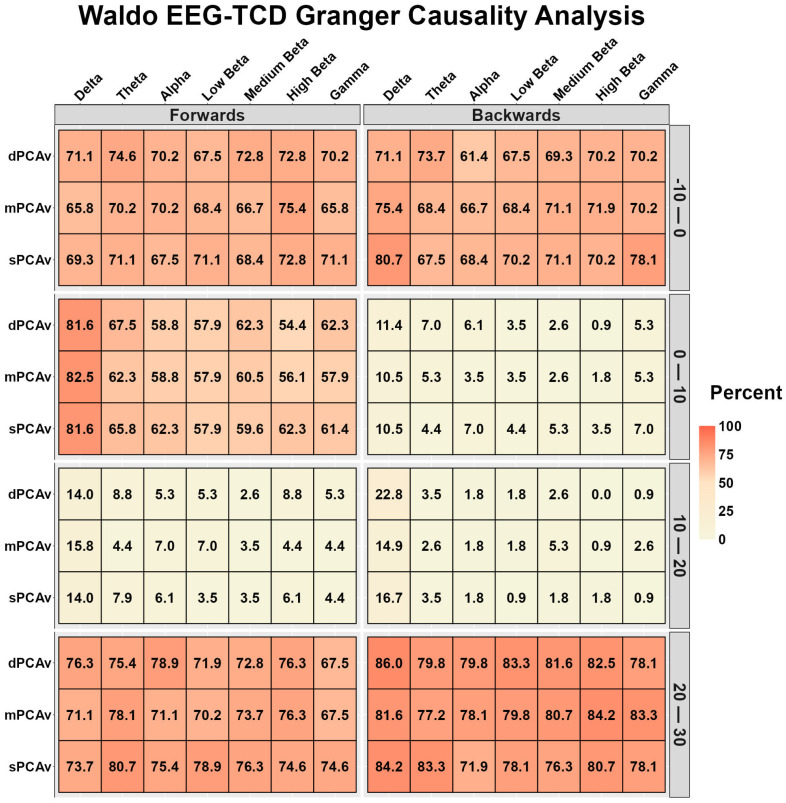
Bidirectional Granger causality analysis results between electroencephalography (EEG) and transcranial Doppler ultrasound (TCD) during the visual “Where’s Waldo?” task. The results are the percentage of participants whose data displayed a significant predictive value between waveforms, stratified into 10-s time bins (*n* = 113, 86 females and 27 males). EEG time–frequency bands are delta (0.5–4 Hz), theta (4–8 Hz), alpha (8–12 Hz), low beta (12–16 Hz), medium beta (16–20 Hz), high beta (20–30 Hz), and gamma (30–40 Hz). Diastolic posterior cerebral artery velocity (dPCAv), mean posterior cerebral artery velocity (mPCAv), systolic posterior cerebral artery velocity (sPCAv).
